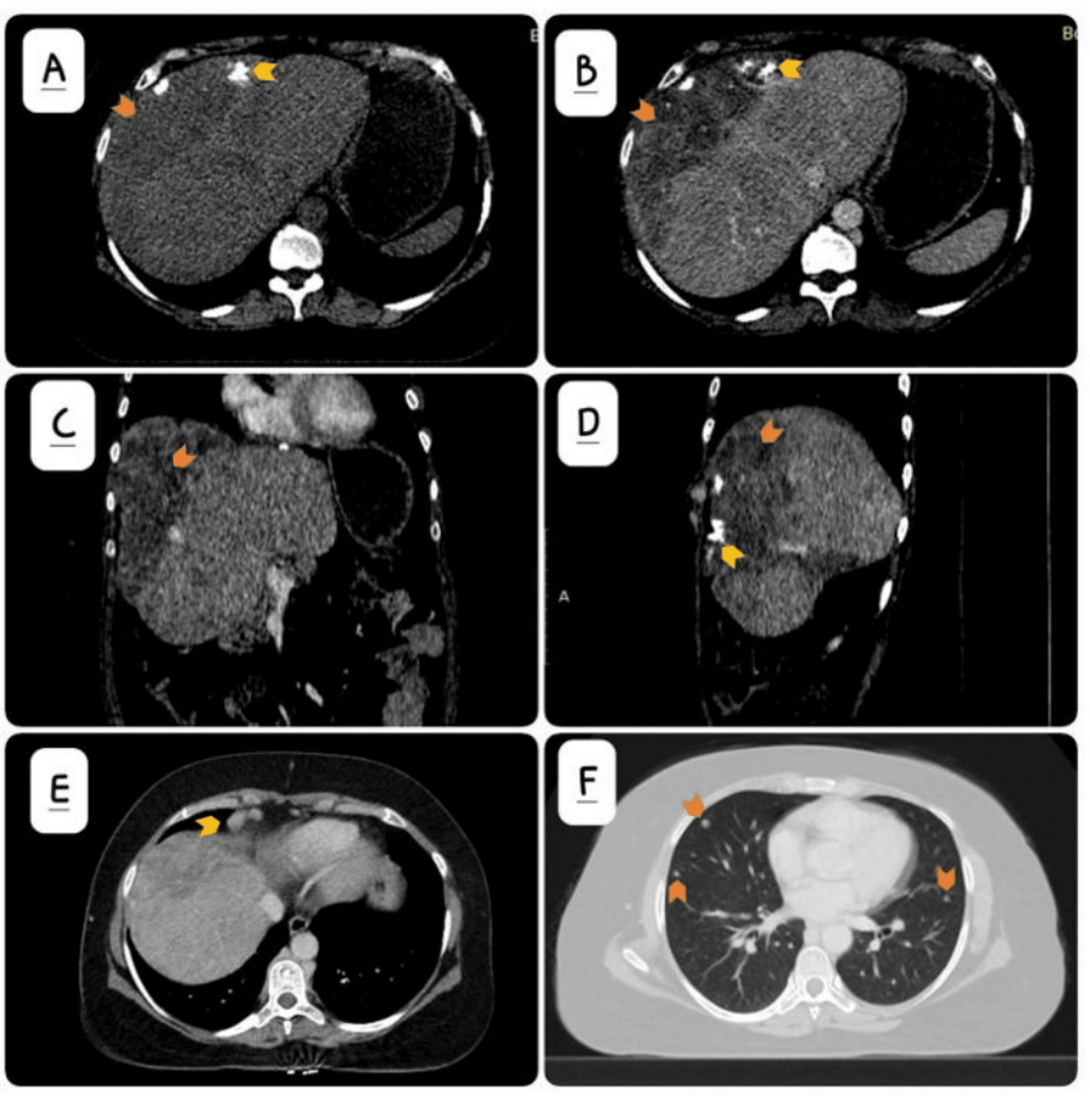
Triphasic contrast-enhanced computed tomography findings A: non-contrast axial CT image demonstrates a large subcapsular confluent hypodense lesion (orange arrow) with chunky calcification (yellow arrow); B: contrast-enhanced axial; C: coronal; and D: sagittal CT images in the portovenous phase show patchy enhancement (orange arrow) and intralesional calcification (yellow arrow); E: contrast-enhanced axial CT image shows enlarged lymph nodes at the right superior diaphragmatic location (yellow arrow); F: soft tissue intrapulmonary nodules (orange arrows) concerning for metastasis.
